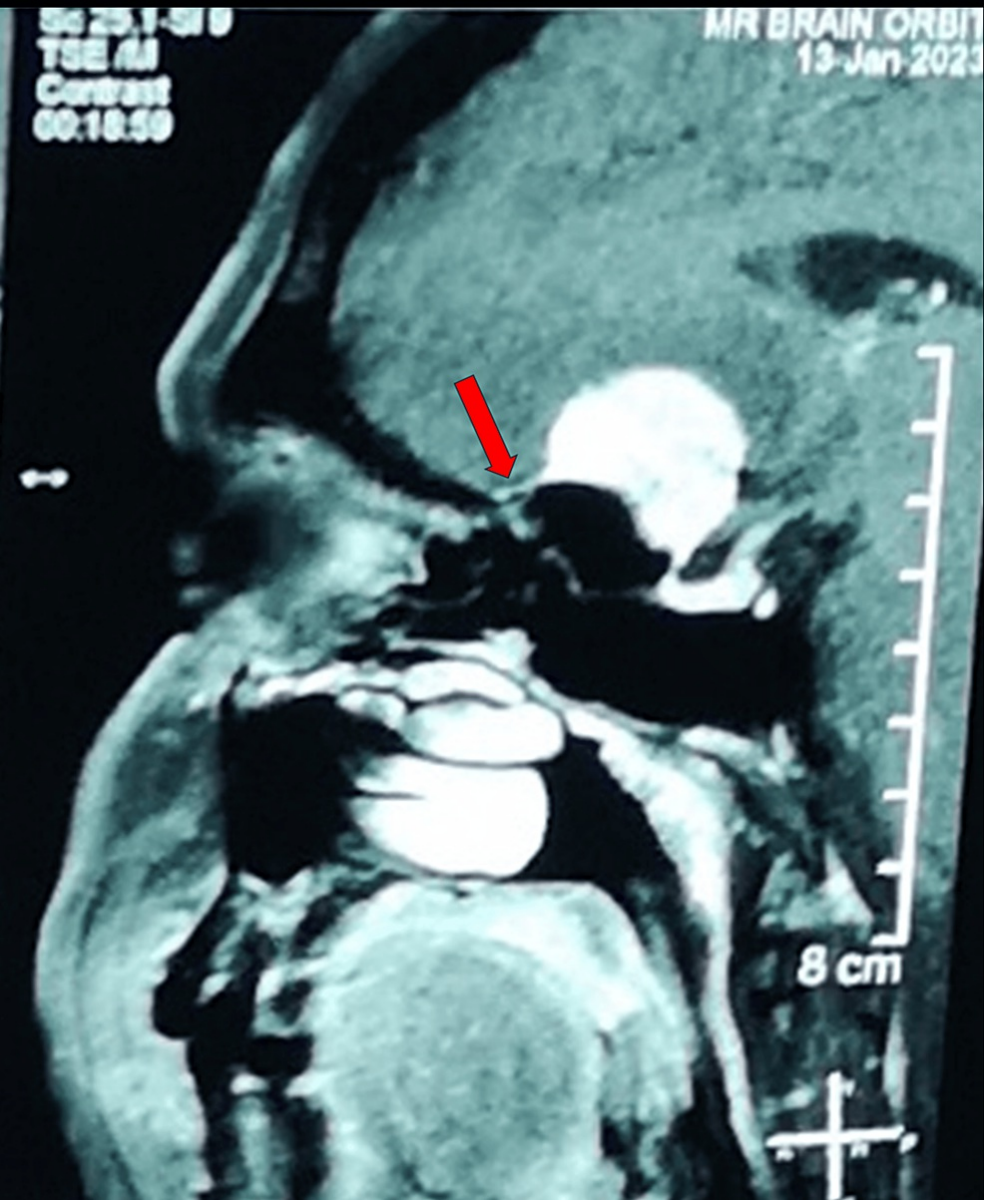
Post-gadolinium MRI T1-weighted image showed hyperintensity enhancement of the suprasellar mass with a dural tail sign (red arrow).
